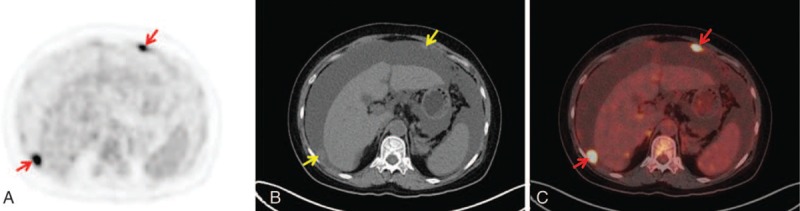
A 64-year-old woman with peritoneal carcinomatosis of colon cancer: focal ^18^F-FDG uptake in the parietal peritoneum (red arrow) on PET and combined PET/CT axial imaging (A and C); nodular abnormalities (yellow arrow) shown on CT axial imaging (B). CT = computed tomography, ^18^F-FDG = F18-fluorodeoxyglucose, PET = positron emission tomography.
